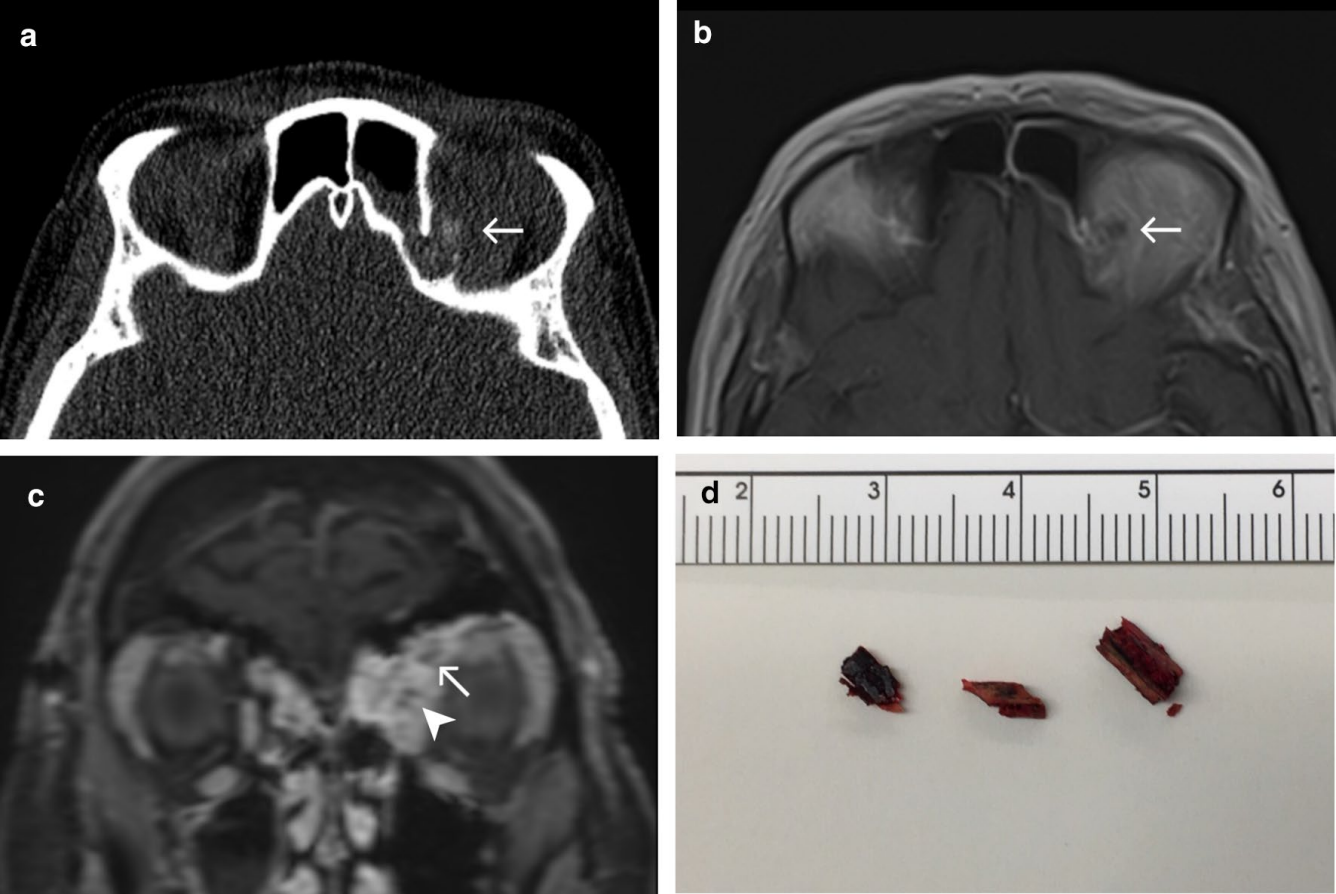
Intraorbital wood. Axial CT image (a) and axial T1-weighted MRI post-contrast (b) demonstrate a small structure close to the orbital roof (arrow), which is moderately hyperdense and hypointense. There is a bony defect in the adjacent wall of the frontal sinus. Coronal T1-weighted MRI with fat saturation post-contrast (c) reveals the aforementioned object (arrow), two more small punctate hypointense foci (arrowheads) and extensive enhancement of the surrounding orbital soft tissues and sinus mucosa. Differential diagnosis for the hypointense structures at this point includes the presence of foreign objects and dislocated bone fragments. Based on MRI alone, abscesses and emphysema should also be considered, but the appearance of a hyperdense object in CT images helps narrow the differential in this case. d A photograph of three wood pieces after their surgical removal
